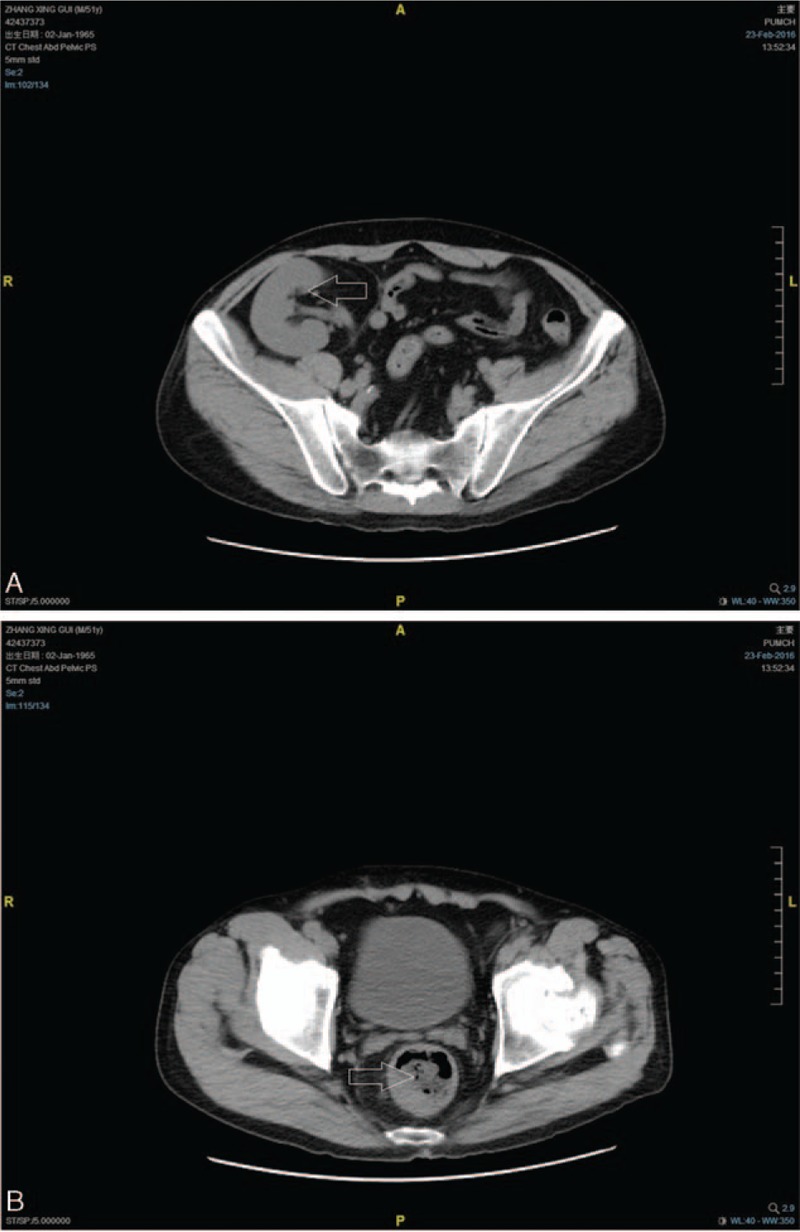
Computed tomography scan showed a soft tissue density mass (arrow) protruding into the lumen of rectum (A) and a transplant kidney (arrow) in the right pelvic cavity (B).
